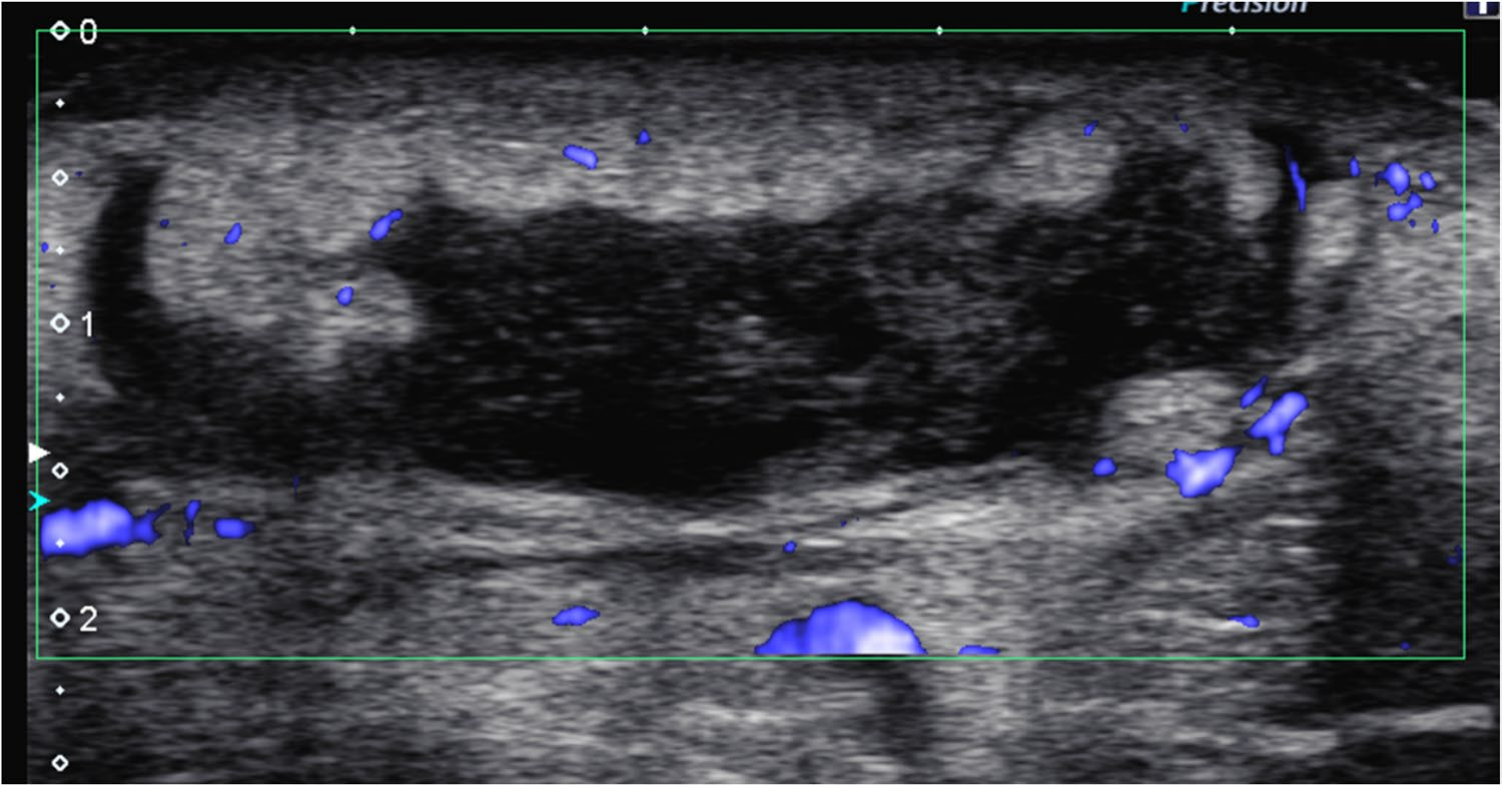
Cellulitis and abscess on ultrasound. A 14-year-old girl who presented to the emergency department with proximal right forearm swelling in the area of bug bites. Power Doppler transverse image of the volar soft tissues of the area shows a large 5-cm hypoechoic collection with peripheral hyperemia. Note the surrounding soft tissues show increased echogenicity
